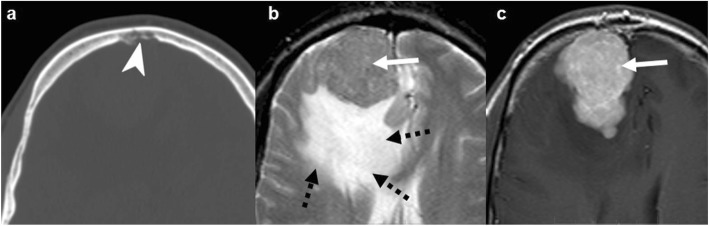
Hemangiopericytoma. CT (a), T2WI (b), and post-contrast T1WI (c). Dural-based “mushroom-like” mass (arrows) with brain edema (dashed-arrows), intense homogeneous enhancement, and foci of bone lysis (arrowhead)
